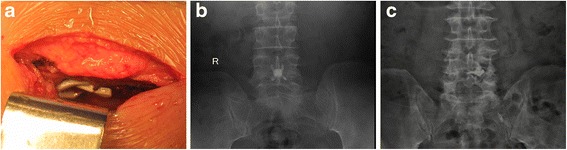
Intraoperative photographs displays that Rocker has been fixed between spinous process (a). Anterior to posterior radiographic image shows a properly placed Rocker (b). Anterior and posterior radiographic image demonstrates that Rocker dislocated to the paraspinous site (c)
